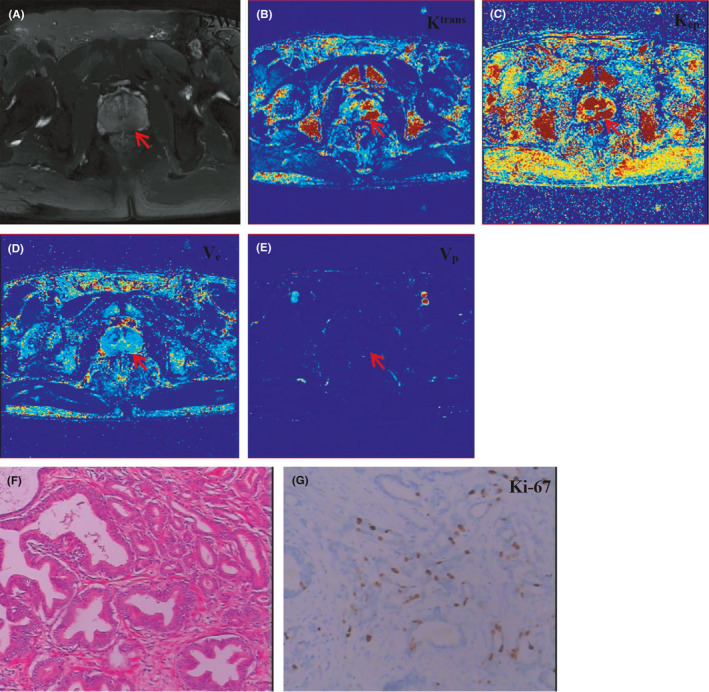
Different imaging modalities demonstrating a single left peripheral prostate cancer from a 62‐year‐old male patient with prostatectomy pathology (Gleason score 3 + 4 and serum PSA 9.89 ng/ml): (A) Transverse T2‐weighted MR image showing a hypointense area with obscure border (red arrow); (B) DCE‐MRI K^trans^ displaying a patchy red region (red arrow); (C) DCE‐MRI K_ep_ displaying a patchy red region (red arrow); (D) DCE‐MRI V_e_ showing a patchy, slightly blue area (red arrow); (E) DCE‐MRI V_p_ showing a dark blue area (red arrow) that is difficult to distinguish; (F) routine pathology (10 × 10); (G) Ki‐67 imaging with 10% positive tumor cells (10 × 10)
